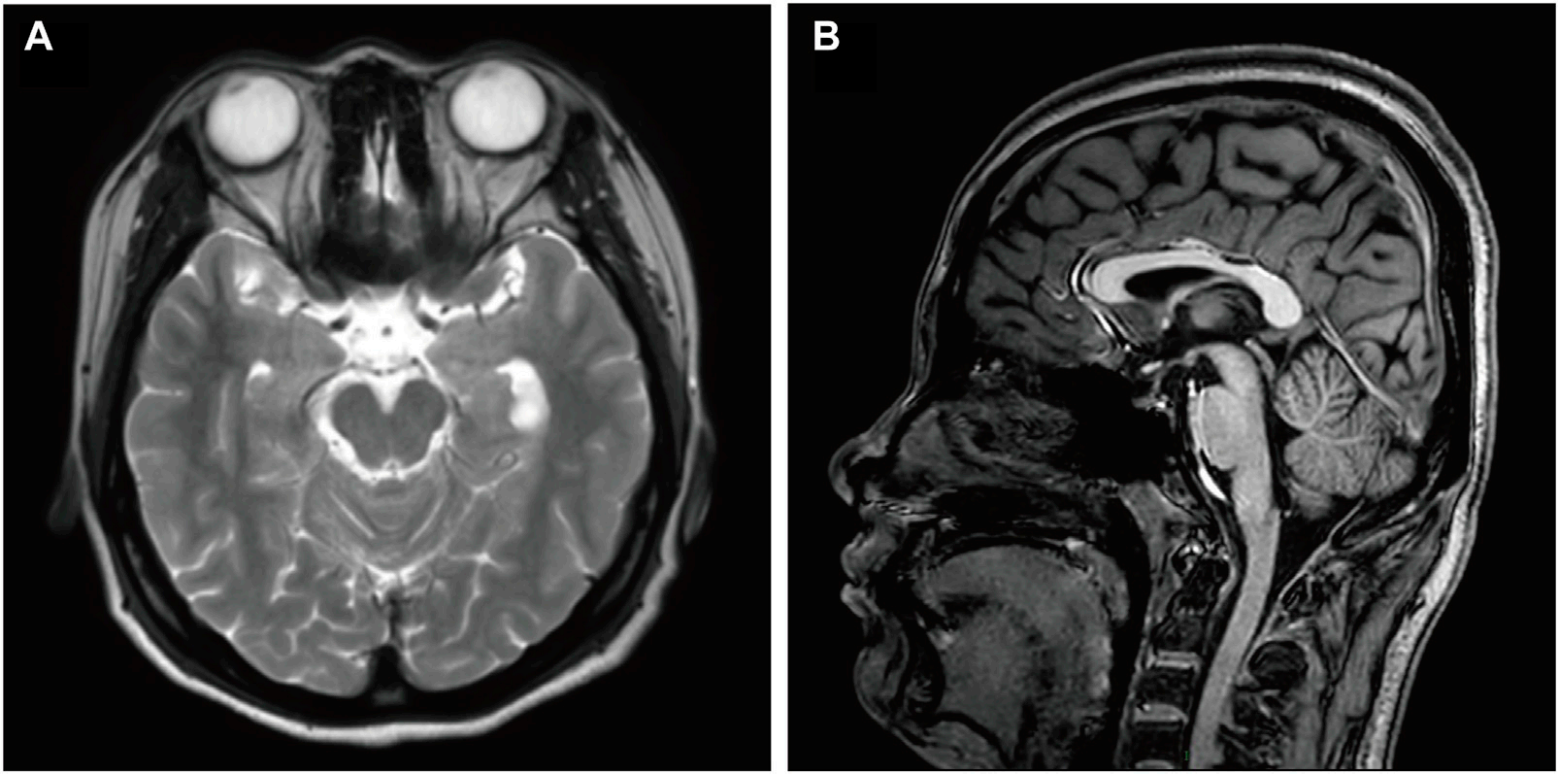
Brain MRI of the described case. (A) Axial T2 and (B) sagittal T1 brain MRI images of this patient, at an age of 22 years. Image A shows a compressed left hippocampus. Image B shows no cerebellar atrophy. MRI, magnetic resonance imaging; FLAIR, fluid-attenuated inversion recovery.
